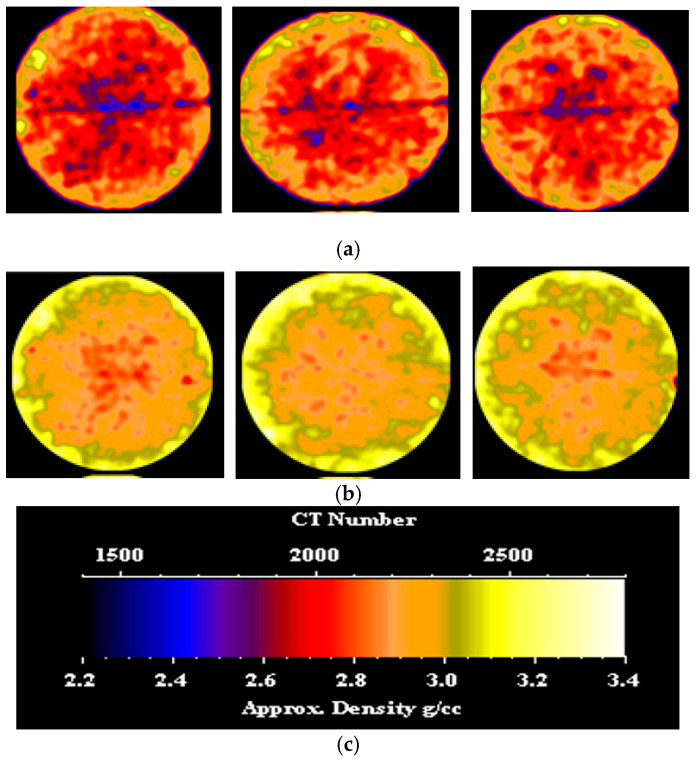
(a) CT scan of the fractured sample before nano-silica gel treatment; (b) CT scan of the fractured sample after nano-silica gel treatment; (c) color code scale.
